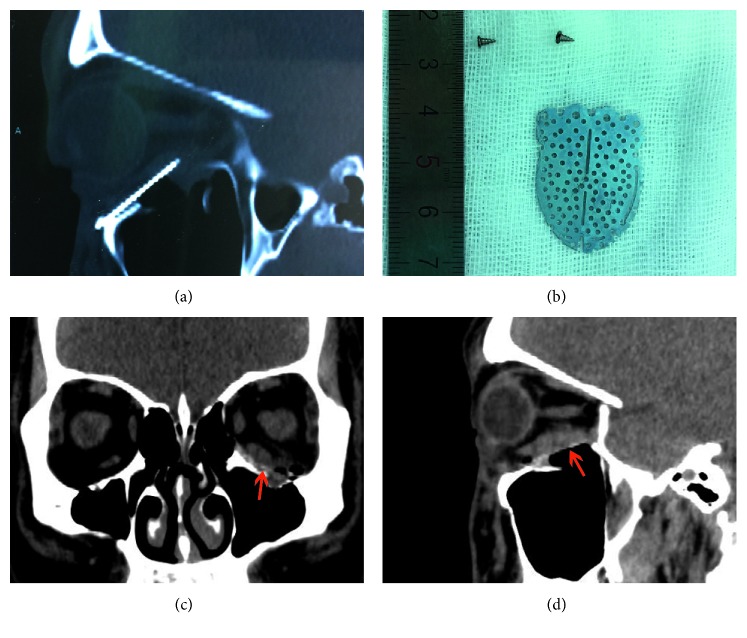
Case with severe ocular movement pain. (a) CT image showing the superiorly displaced floor implant and significantly incarcerated extraocular muscle. (b) The removed titanium mesh and screws. (c and d) Coronal and sagittal CT views of the implanted RapidSorb at 10 months postoperatively.
